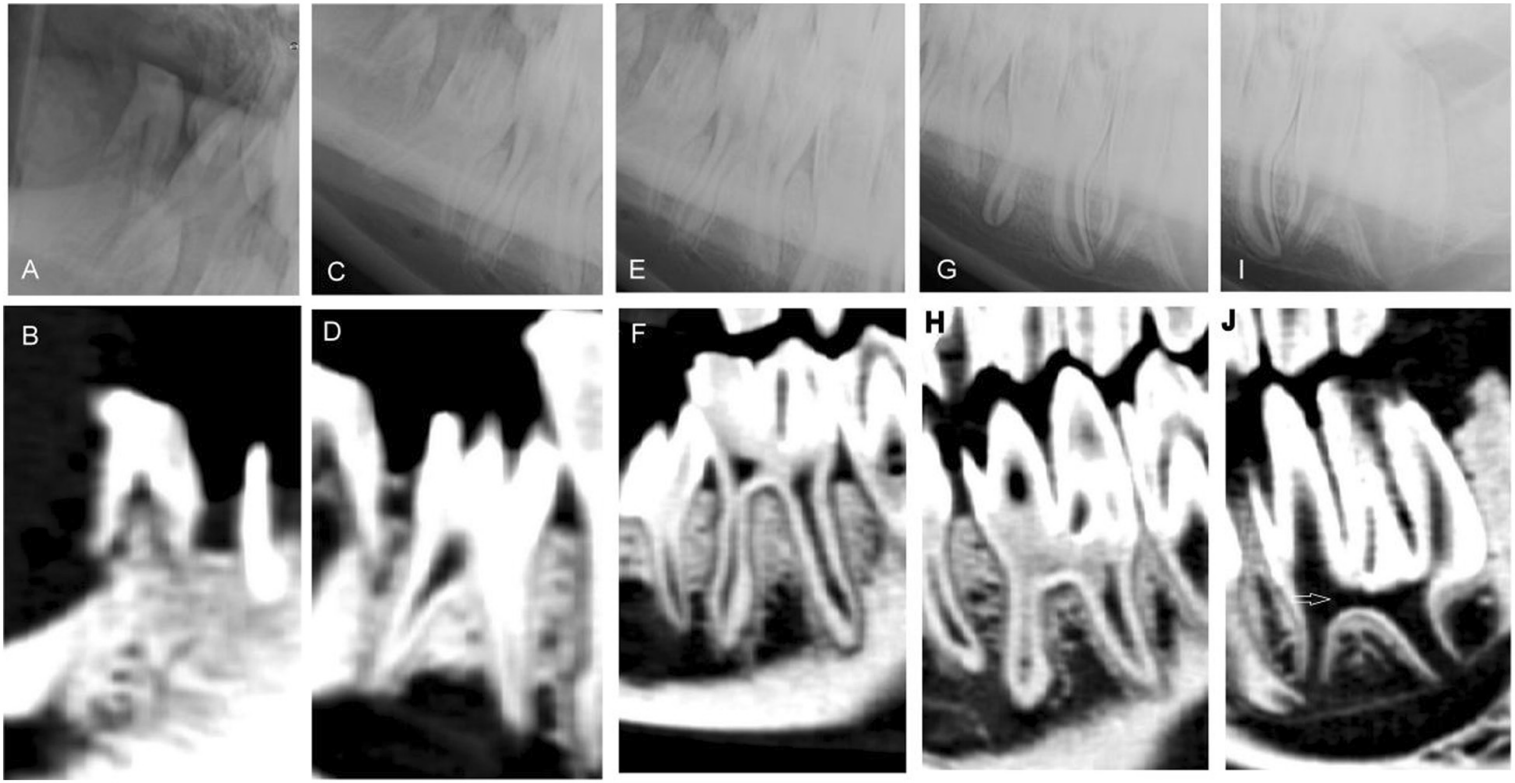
Mandibular cheek teeth. Mandibular cheek teeth of an adult llama (age 44 months). There were two premolars and three molars on each hemi-mandible. A/C/E/G/I: Left to right lateral30°ventral-laterodorsal oblique projection showing the right mandible. B/D/F/H/J: Sagittal CT-reconstructions of mandibular 07 to 11. A and B: 07, C and D: 08, E and F: 09, G and H: 10, I and J: 11. J shows a stage, where the common pulp chamber is still existing (white arrow).
